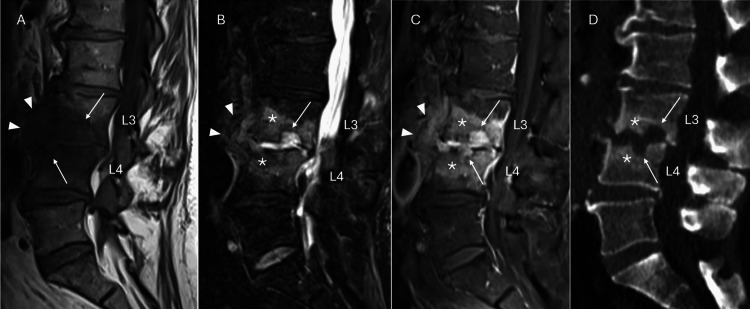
Sagittal lumbar spine sequences: A) MRI T1W, B) MRI T2W with fat suppression, C) MRI T1W with fat suppression and gadolinium injection, and D) sagittal reconstruction of lumbar spine CT scan. MRI showed bone marrow edema at L3-L4 (stars), adjacent to endplate erosions (arrows). The erosions and intervertebral space were filled with highly hydrated tissue (marked hyperintensity in T2W images [B]), which was highly enhanced after gadolinium injection (C), corresponding to granulation tissue, with prevertebral swelling and infiltration (head arrows). In the CT scan (D), the erosions were deep with blurred margins (arrows), associated with reactive sclerosis of the trabecular bone (stars). MRI, magnetic resonance imaging; T1W, T1-weighted; T2W, T2-weighted; CT, computed tomography
